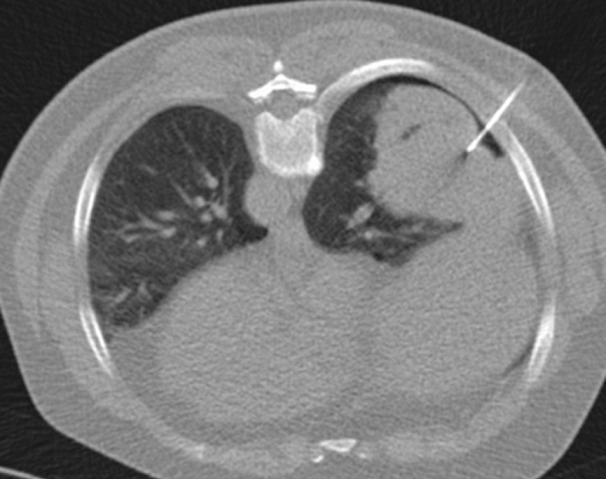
Axial CT image showing post procédural pneumothorax in 65 years old man who underwent core needle biopsy of right postéro basal mass
